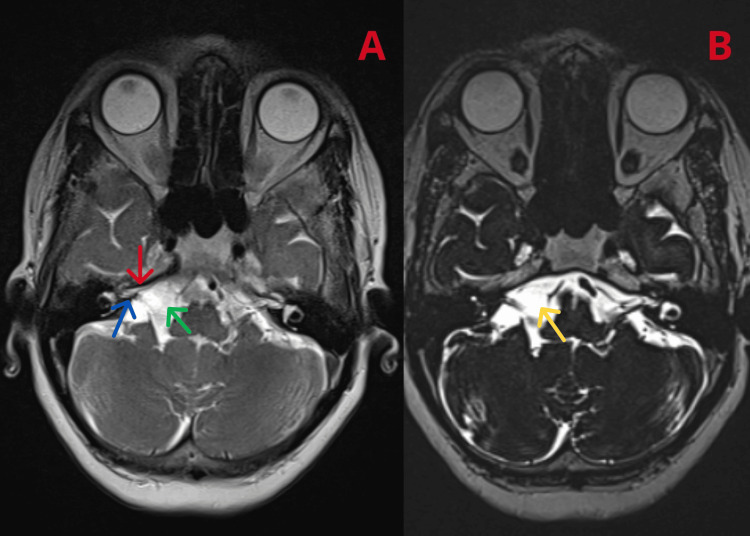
MRI of the brain with MRA and MRV showing (A) CSF signal intensity area in the right CP angle (~2.1 × 1.2 × 0.9 cm) causing a widening of the lateral cerebellar medullary cistern abutting the seventh (red arrow) and eighth (blue arrow) cranial nerves, its inferior aspect, and AICA branches diagnostic of arachnoid cyst (green arrow in A and yellow arrow in B). MRI: magnetic resonance imaging; MRA: magnetic resonance angiography; MRV: magnetic resonance venography; AICA: anterior inferior cerebellar artery; CSF: cerebrospinal fluid; CP: cerebellopontine
